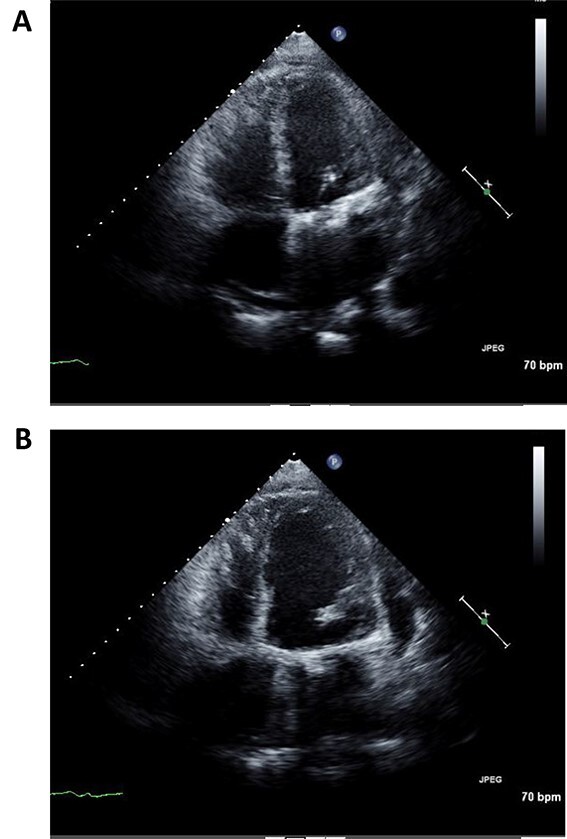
Postoperative echocardiography of left ventricle in systolic (A) and diastolic (B) phases.
